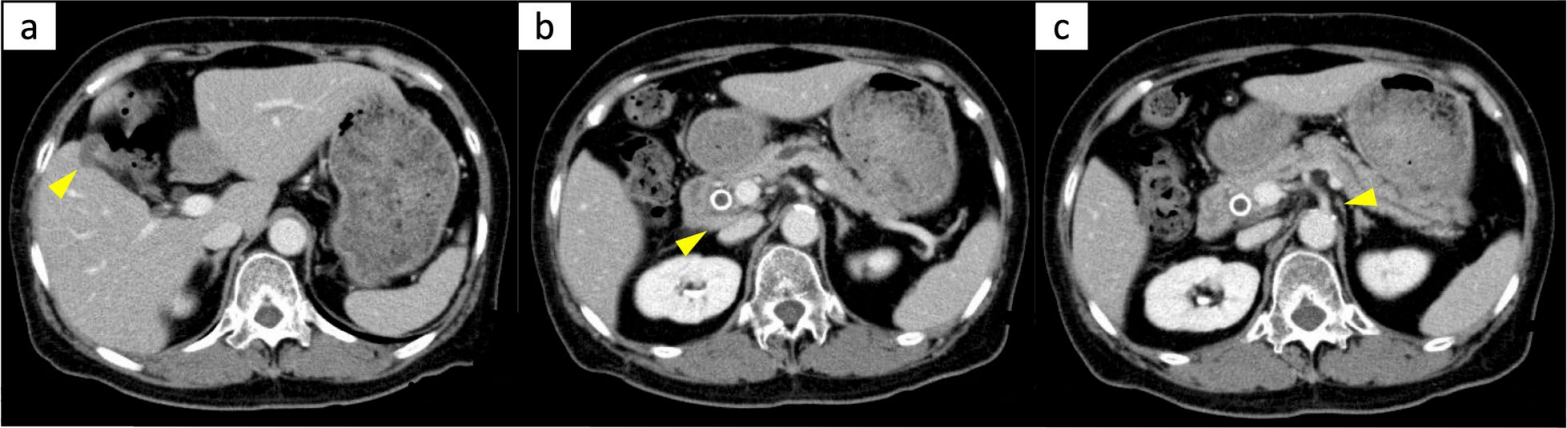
Multidetector-row computed tomography findings after two courses of gemcitabine plus cisplatin therapy. a The shrunken tumor at the fundus of the gallbladder. b The shrunken metastasis lymph node in the hepatoduodenal mesentery. c The shrunken para-aortic lymph node
